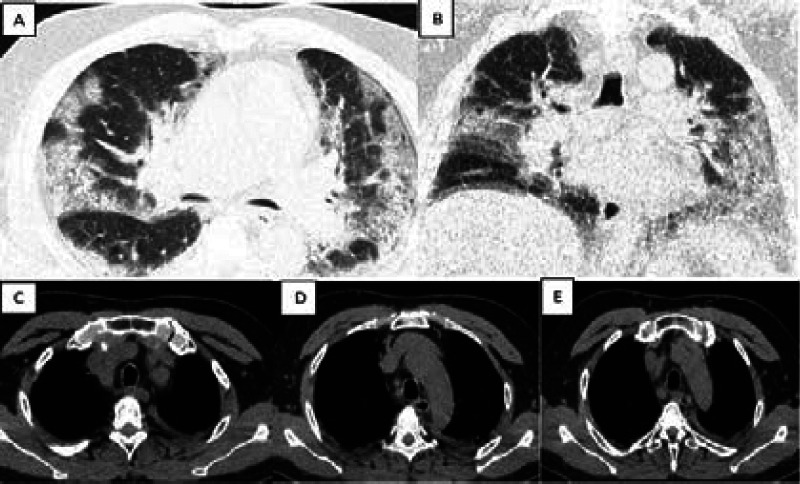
67-year-old with Diabetes and Hypertension, negative RT PCR who presented with difficulty in breathing for 5 days. Axial (, A, B) Chest HRCT shows diffuse GOO with a peripheral predominance. Axial Chest CT scans (C, D, E) show superior mediastinal lymphnodes. He was classified as CORADS 5, stage 3, with a severity score of 20/25
